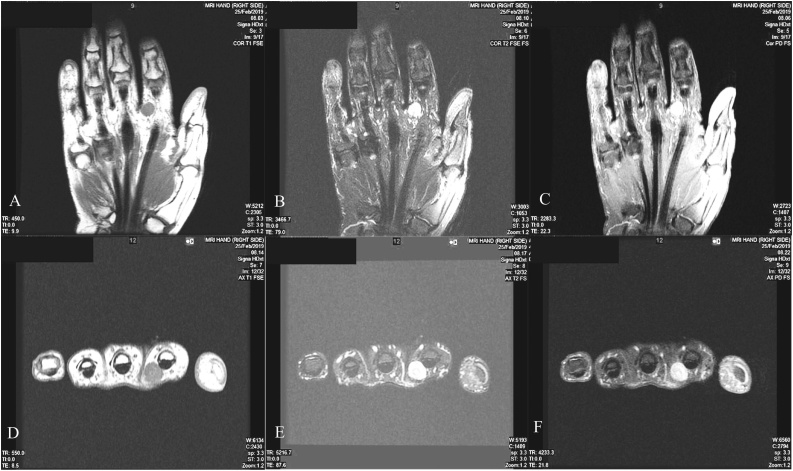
MRI of the right hand taken on different views and modes showing the mass. A: Coronal T1; B: Coronal T2; C: Coronal PD; D: Axial T1; E: Axial T2; F: Axial PD.
